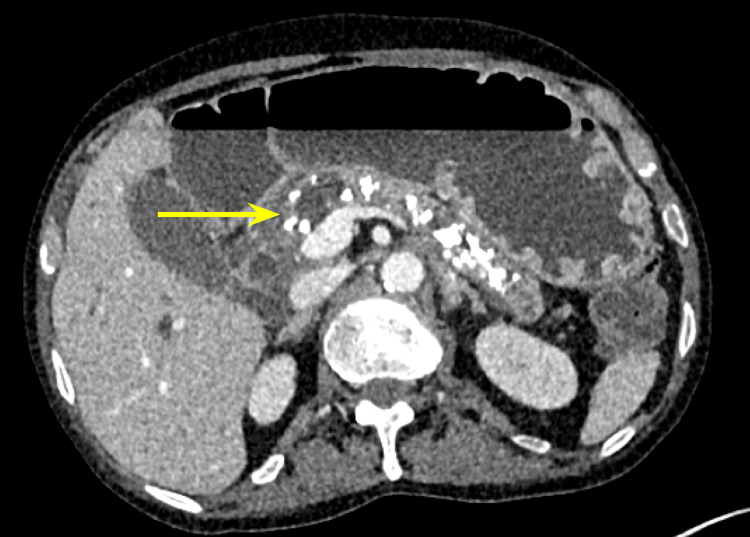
Chronic pancreatitis evidenced by heavy calcification on computed tomography of the abdomen and pelvis.
